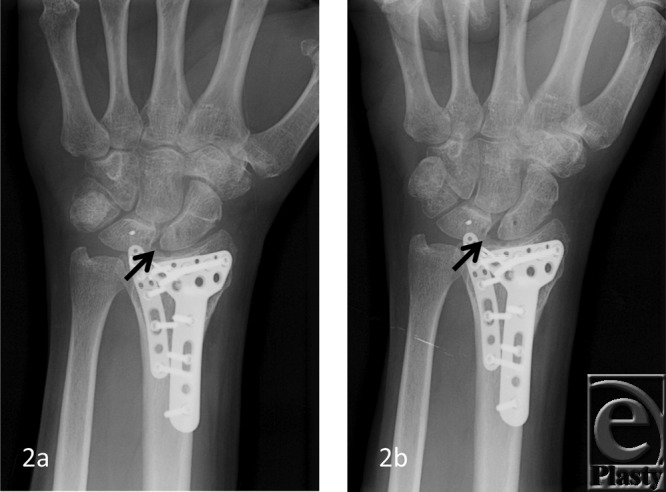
A 62-year-old man with prior ORIF and SLIL ligament repair. Normal SL space (*arrow*) on posteroanterior X-ray view (*a*). Notice mild gaping of S-L interval (*arrow*) on clenched fist posteroanterior X-ray view (*b*). ORIF indicates open reduction internal fixation; SLIL, scapholunate interosseus ligament.
